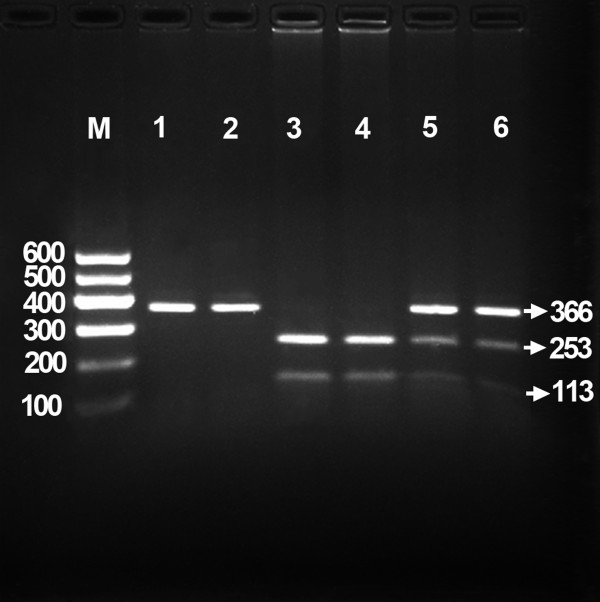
Genotyping of the *MLXIPL/TBL2 *rs17145738 SNP. Lane M: 100 bp marker ladder; lanes 1 and 2: TT genotype (366 bp); lanes 3 and 4: CC genotype (253- and 113-bp); and lanes 5 and 6: CT genotype (366-, 253-, and 113-bp). The 49-bp fragments were invisible in the gel owing to its fast migration speed.
